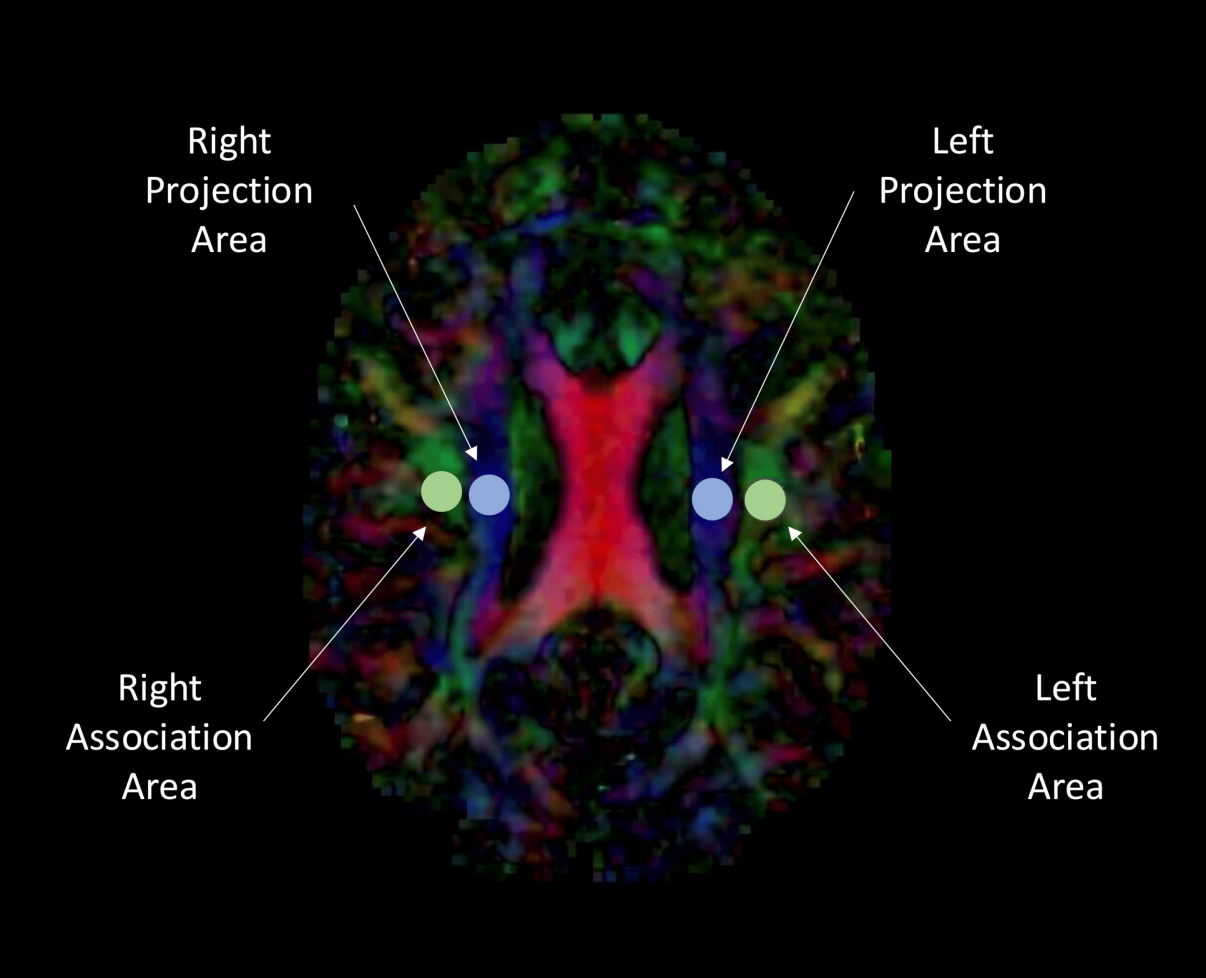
ROI placement for DTI analysis Axial MRI of the head demonstrating diffusion tensor tracks. ROIs are placed in the left and right hemispheres in the projection and association areas for DTI-ALPS index calculation. DTI, diffusion tensor imaging; DTI-ALPS, diffusion tensor imaging analysis along the perivascular space; ROI, region of interest The image was generated using 3D Slicer software, Version 5.8.1 (3D Slicer, Earth, TX, USA).
